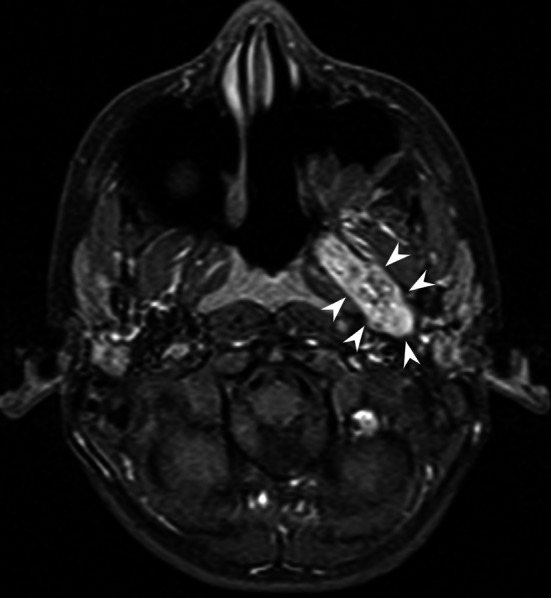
Axial contrast-enhanced T1-weighted MR image with fat saturation demonstrates residual tumor between the pterygoid plates (*arrowheads*)
